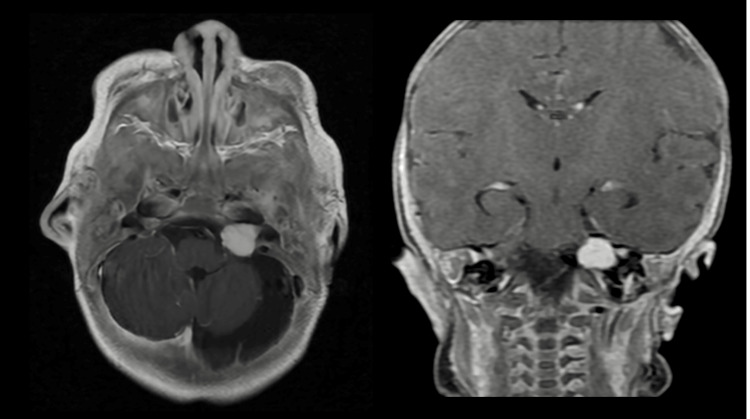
Brain MRI with contrast showed a 1.1 cm contrast-enhancing mass in the left cerebellopontine angle, suggestive of a vestibular schwannoma. This finding correlates with the patient’s failed newborn hearing screening, raising concerns about congenital auditory dysfunction unrelated to the typical vascular anomalies seen in PHACE syndrome.
